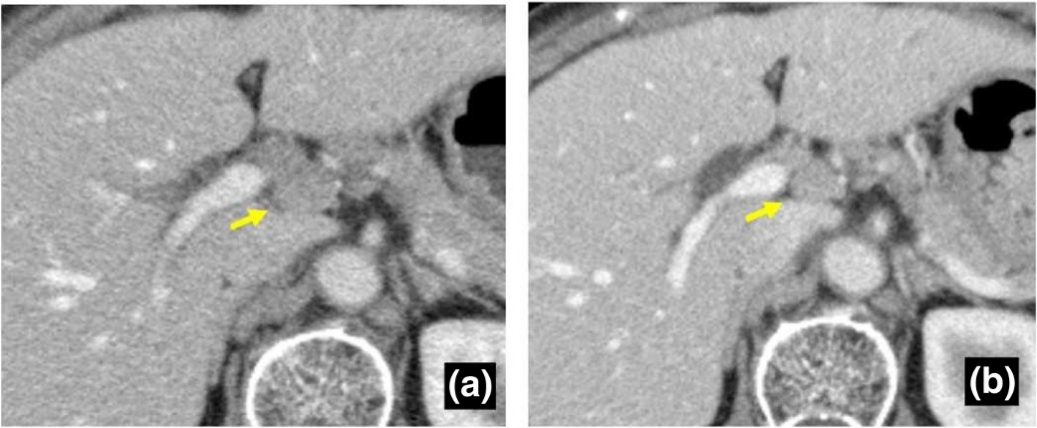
Course of remaining swollen lymph nodes. a) CT 5 months after surgery shows a remaining swollen lymph node (No. 12). The size is 15 × 11 mm. b) CT 46 months after surgery also shows the remaining swollen No. 12 lymph node. The size (11 × 8 mm) appears to be reduced compared to the initial examination.
